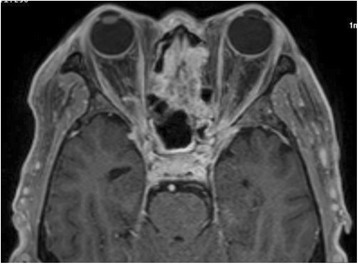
Contrast-enhanced magnetic resonance imaging (MRI), axial view. A strongly enhanced lesion was observed in the nasal septum, ethmoid sinuses, bilateral orbital apexes, and cavernous sinus
